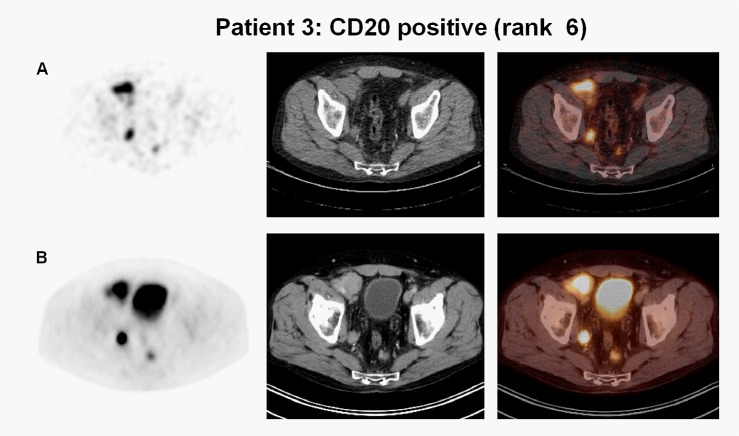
Example of tumor uptake on ^89^Zr-rituximab-PET, concordant with CD20 expression in biopsy. Axial images, from left to right attenuation corrected PET, low dose CT and fused PET/CT image of patient 3. a) ^89^Zr-rituximab-PET shows intense tumor uptake concordant with a CD20-positive biopsy (inguinal lymph node). b) Corresponding tumor location on ^18^F-FDG-PET.
